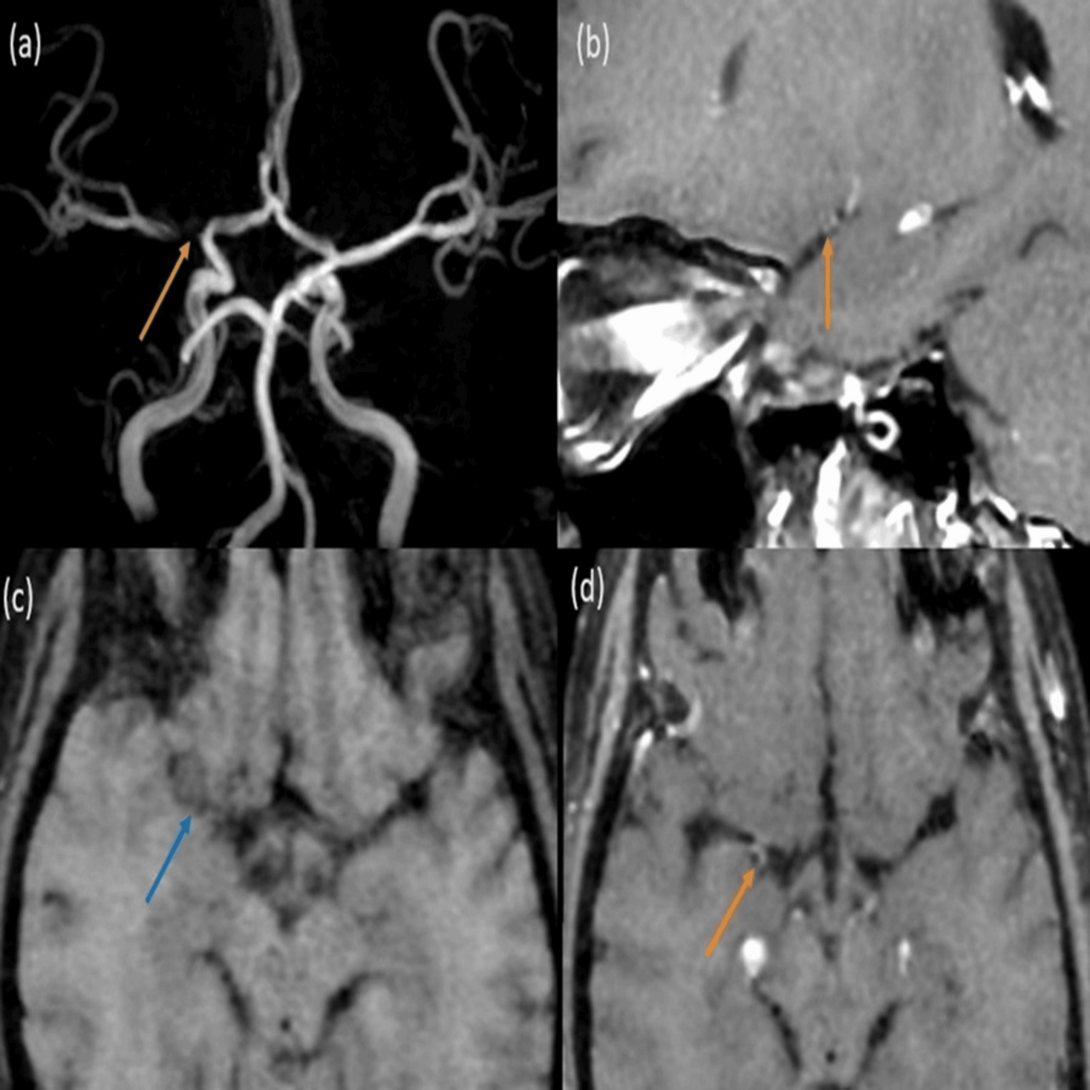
CNS vasculitis: (a) TOF MRA images demonstrating occlusion of the right proximal M1 MCA segment (orange arrow). (b and d) VW-MRI sagittal and post-contrast axial T1 BB sequences demonstrating circumferential irregular wall thickening and enhancement (orange arrows) involving the right proximal M1 MCA segment with its complete occlusion. (c) There is also the presence of non-enhancing dirty CSF in the right Sylvian cistern likely due to basal exudates (blue arrow). Diagnosis of granulomatous tuberculous vasculitis is considered. CNS: central nervous system; TOF MRA: time-of-flight magnetic resonance angiography; VW-MRI: vessel wall magnetic resonance imaging; BB: black blood; MCA: middle cerebral artery; CSF: cerebrospinal fluid
